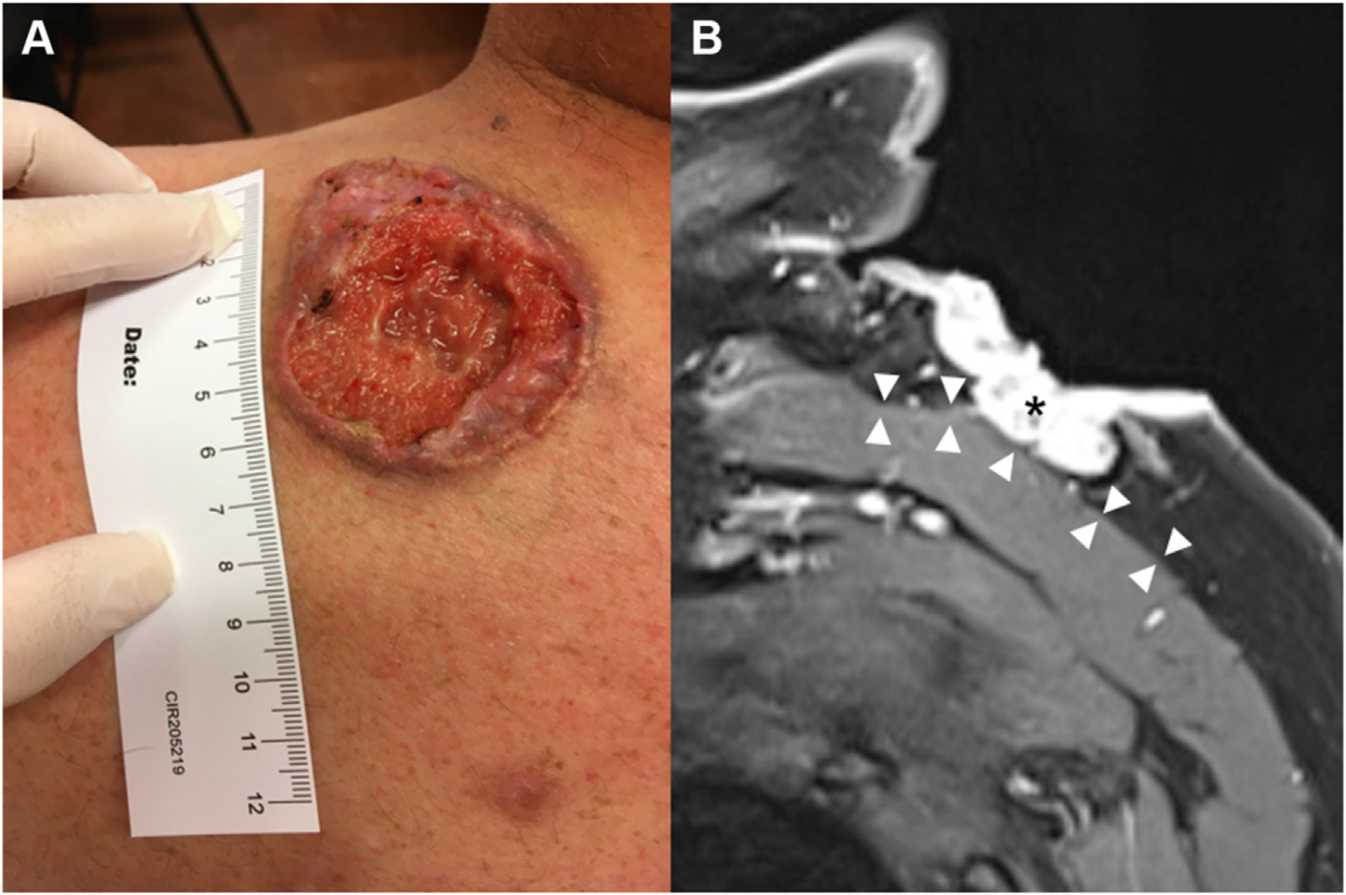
A, Clinical picture of a giant basal cell carcinoma (BCC) of the left shoulder. B, Sagittal view of a contrast-enhanced T1-weighted magnetic resonance imaging of the left shoulder, showing contrast enhancement in the BCC (*black asterisk*) and in the fascia of the trapezius muscle (delineated by *whitearrowheads*).
